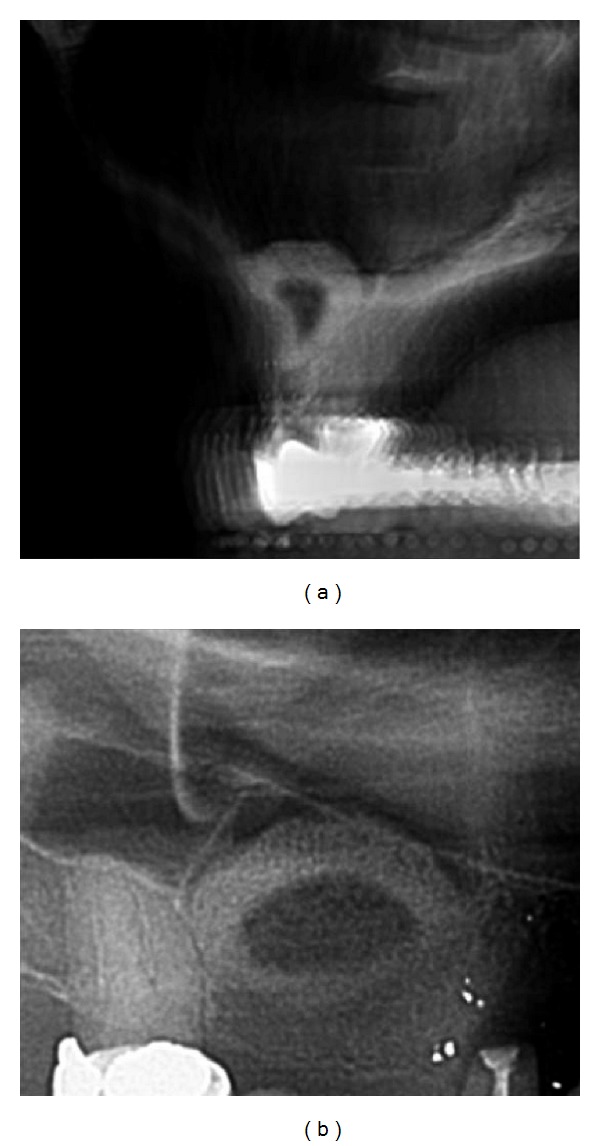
Case 3: transversal and parasagittal CBCT scan 4 months after tHUCSL-Intralift. The even circular centripetal calcification process can be observed.
